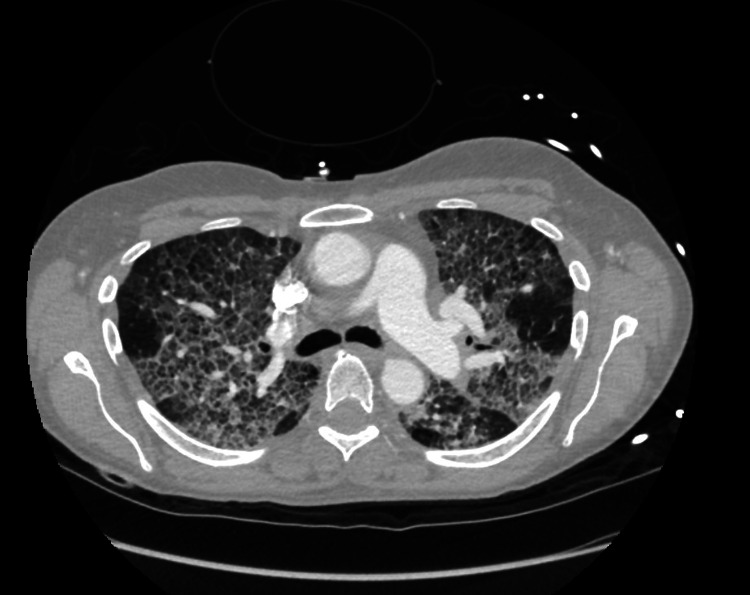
Chest CT scan findings with crazy-paving pattern with interlobular septal thickening
